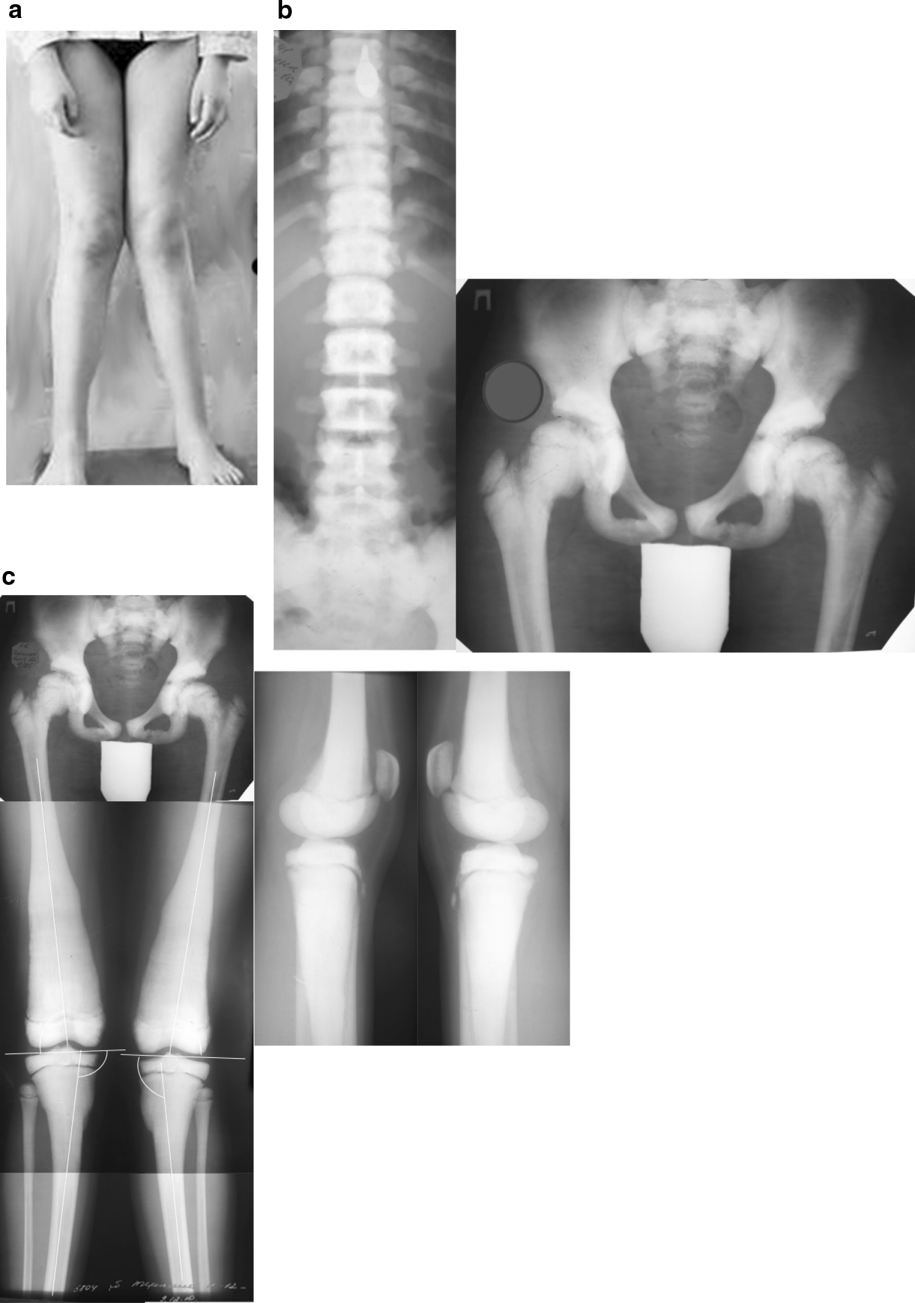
Before surgery: a aspect of lower limbs; b AP radiographs of the spine and pelvis, note increased bone density; c radiographs of lower limbs: aLDFA 84° (*right side*) and 82° (*left side*); aMPTA 97° for both tibias
